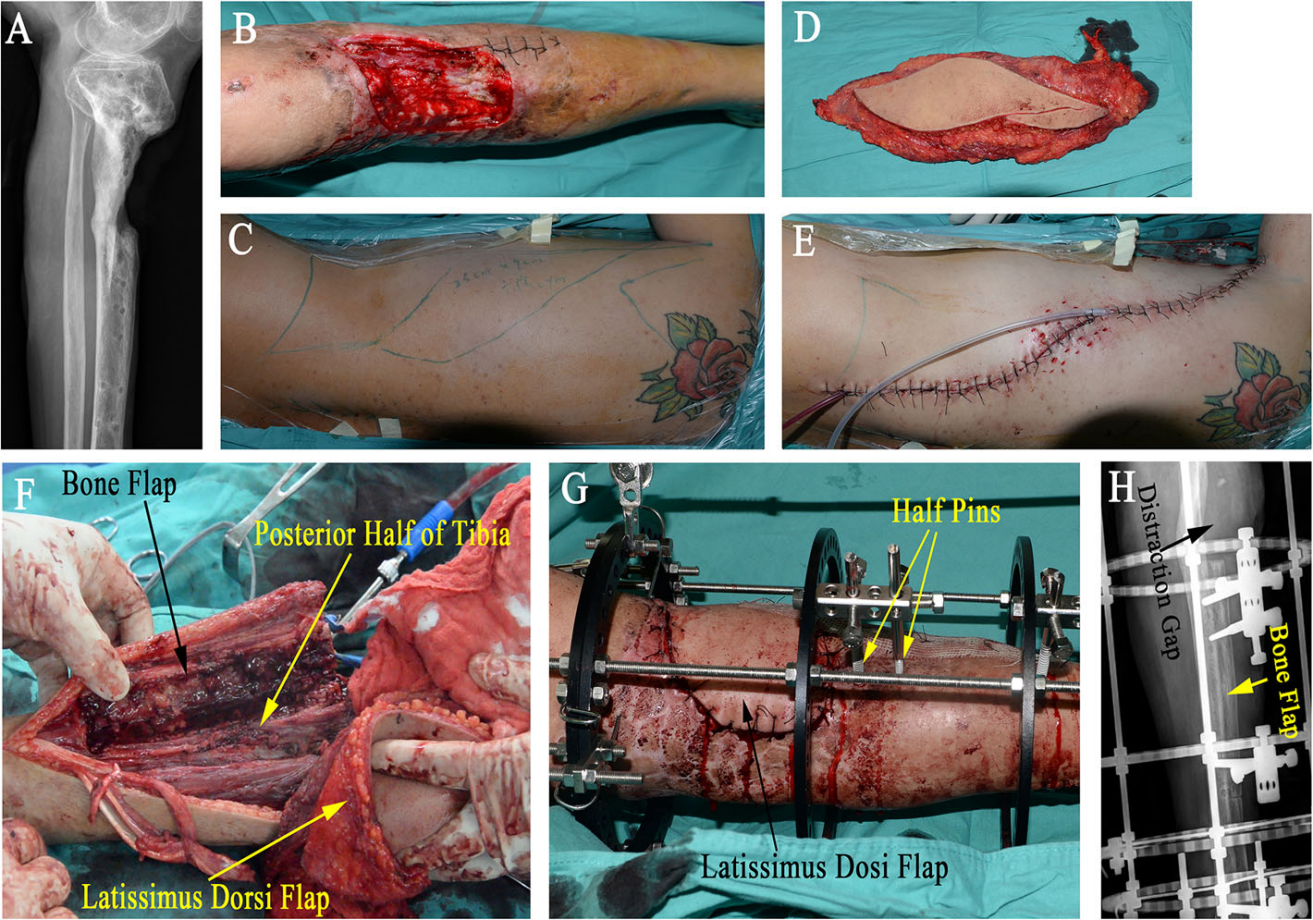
A 33-year-old man with chronic osteomyelitis of the left tibia. a Radiograph shows bone defect following resection of dead bone. b Soft tissue defect following debridement. c Planning on the harvest of free latissimus dorsi flap. d The latissimus dorsi flap is harvested. e The donor site is sutured directly drainage tubes. f The bone flap is separated from the posterior half of tibia, and one tip of the flap is sewed up with the wound edge. g The latissimus dorsi flap completely covers the soft tissue defect, the external fixator is assembled, and two half pins are used to fix the bone flap. h Immediate postoperative lateral radiograph shows distraction gap and bone flap
